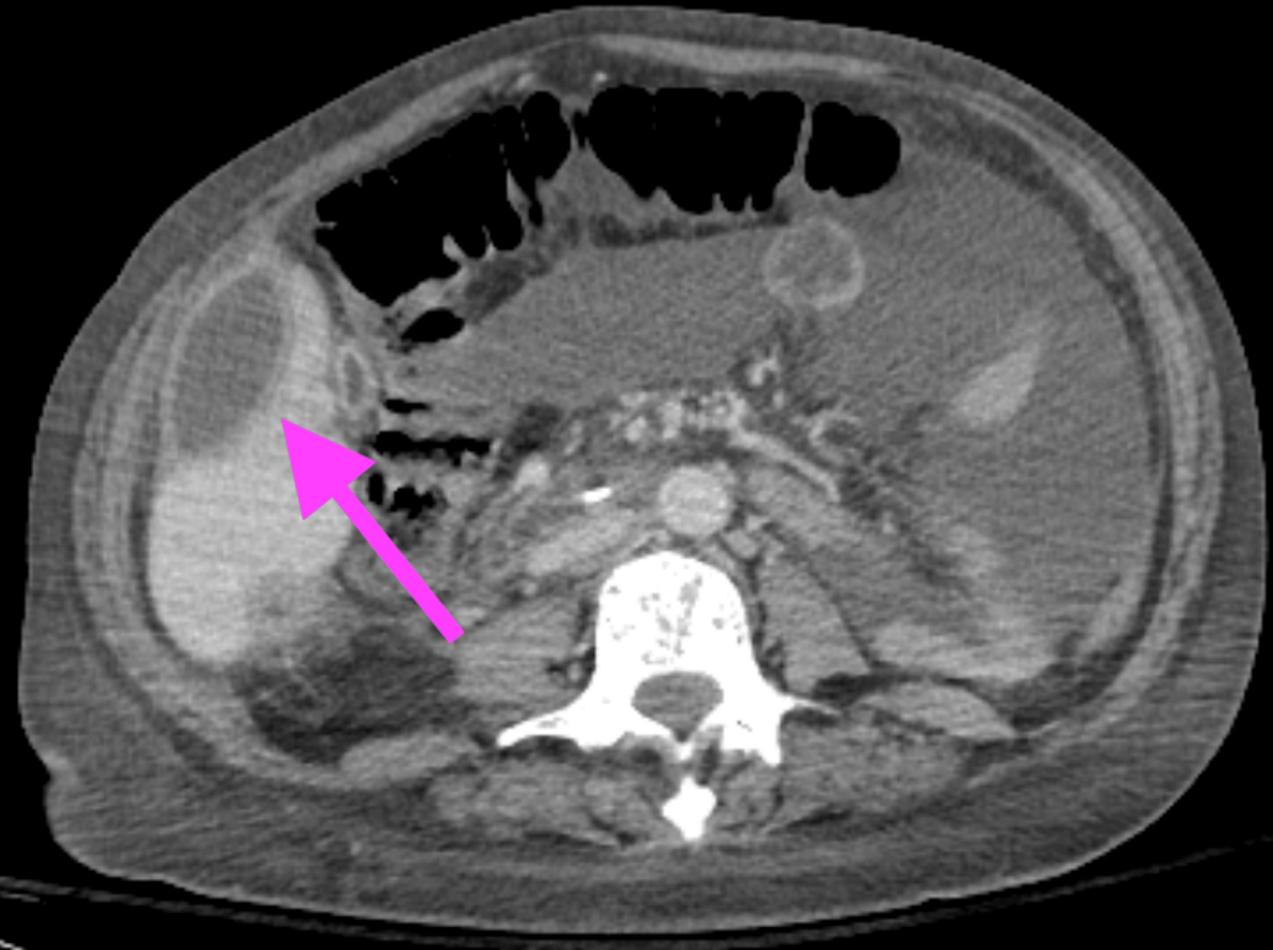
A repeat CT scan of the abdomen and pelvis showed interval development of a rim-enhancing abscess abutting the right hepatic lobe extending inferiorly into the pelvis, measuring 17 cm in diameter, and an interval development of a ring-enhancing abscess in the left subphrenic space, measuring about 7 cm. The arrow highlights intrahepatic abscess.
